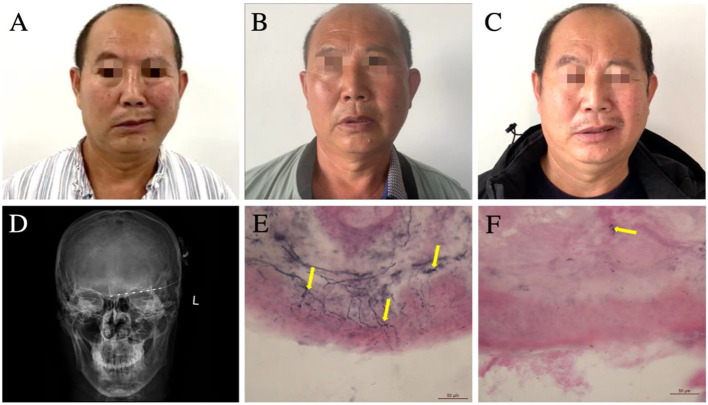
(A–C) Changes in the patient's hemifacial and cervical dystonia over time. (A) Pre-implant surgery, the dystonia on the left side of the patient's face is seen, manifesting as an involuntary contraction of the left hemifacial. (B) Satisfactory relief of the dystonia is still evident at 6 months after PNS surgery. (C) Involuntary contraction of the left-sided facial muscles reappeared at 12 months after PNS surgery. (D) Anteroposterior radiograph of the cranium demonstrating the placement of the supraorbital PNS-stimulating electrodes. (E, F) Bilateral forehead skin biopsy using immunolabeled protein gene product 9.5 demonstrating markedly lower epidermal neurite (yellow arrows) density in the left forehead (F) vs. that in the right forehead (E). PNS, peripheral nerve stimulation.
